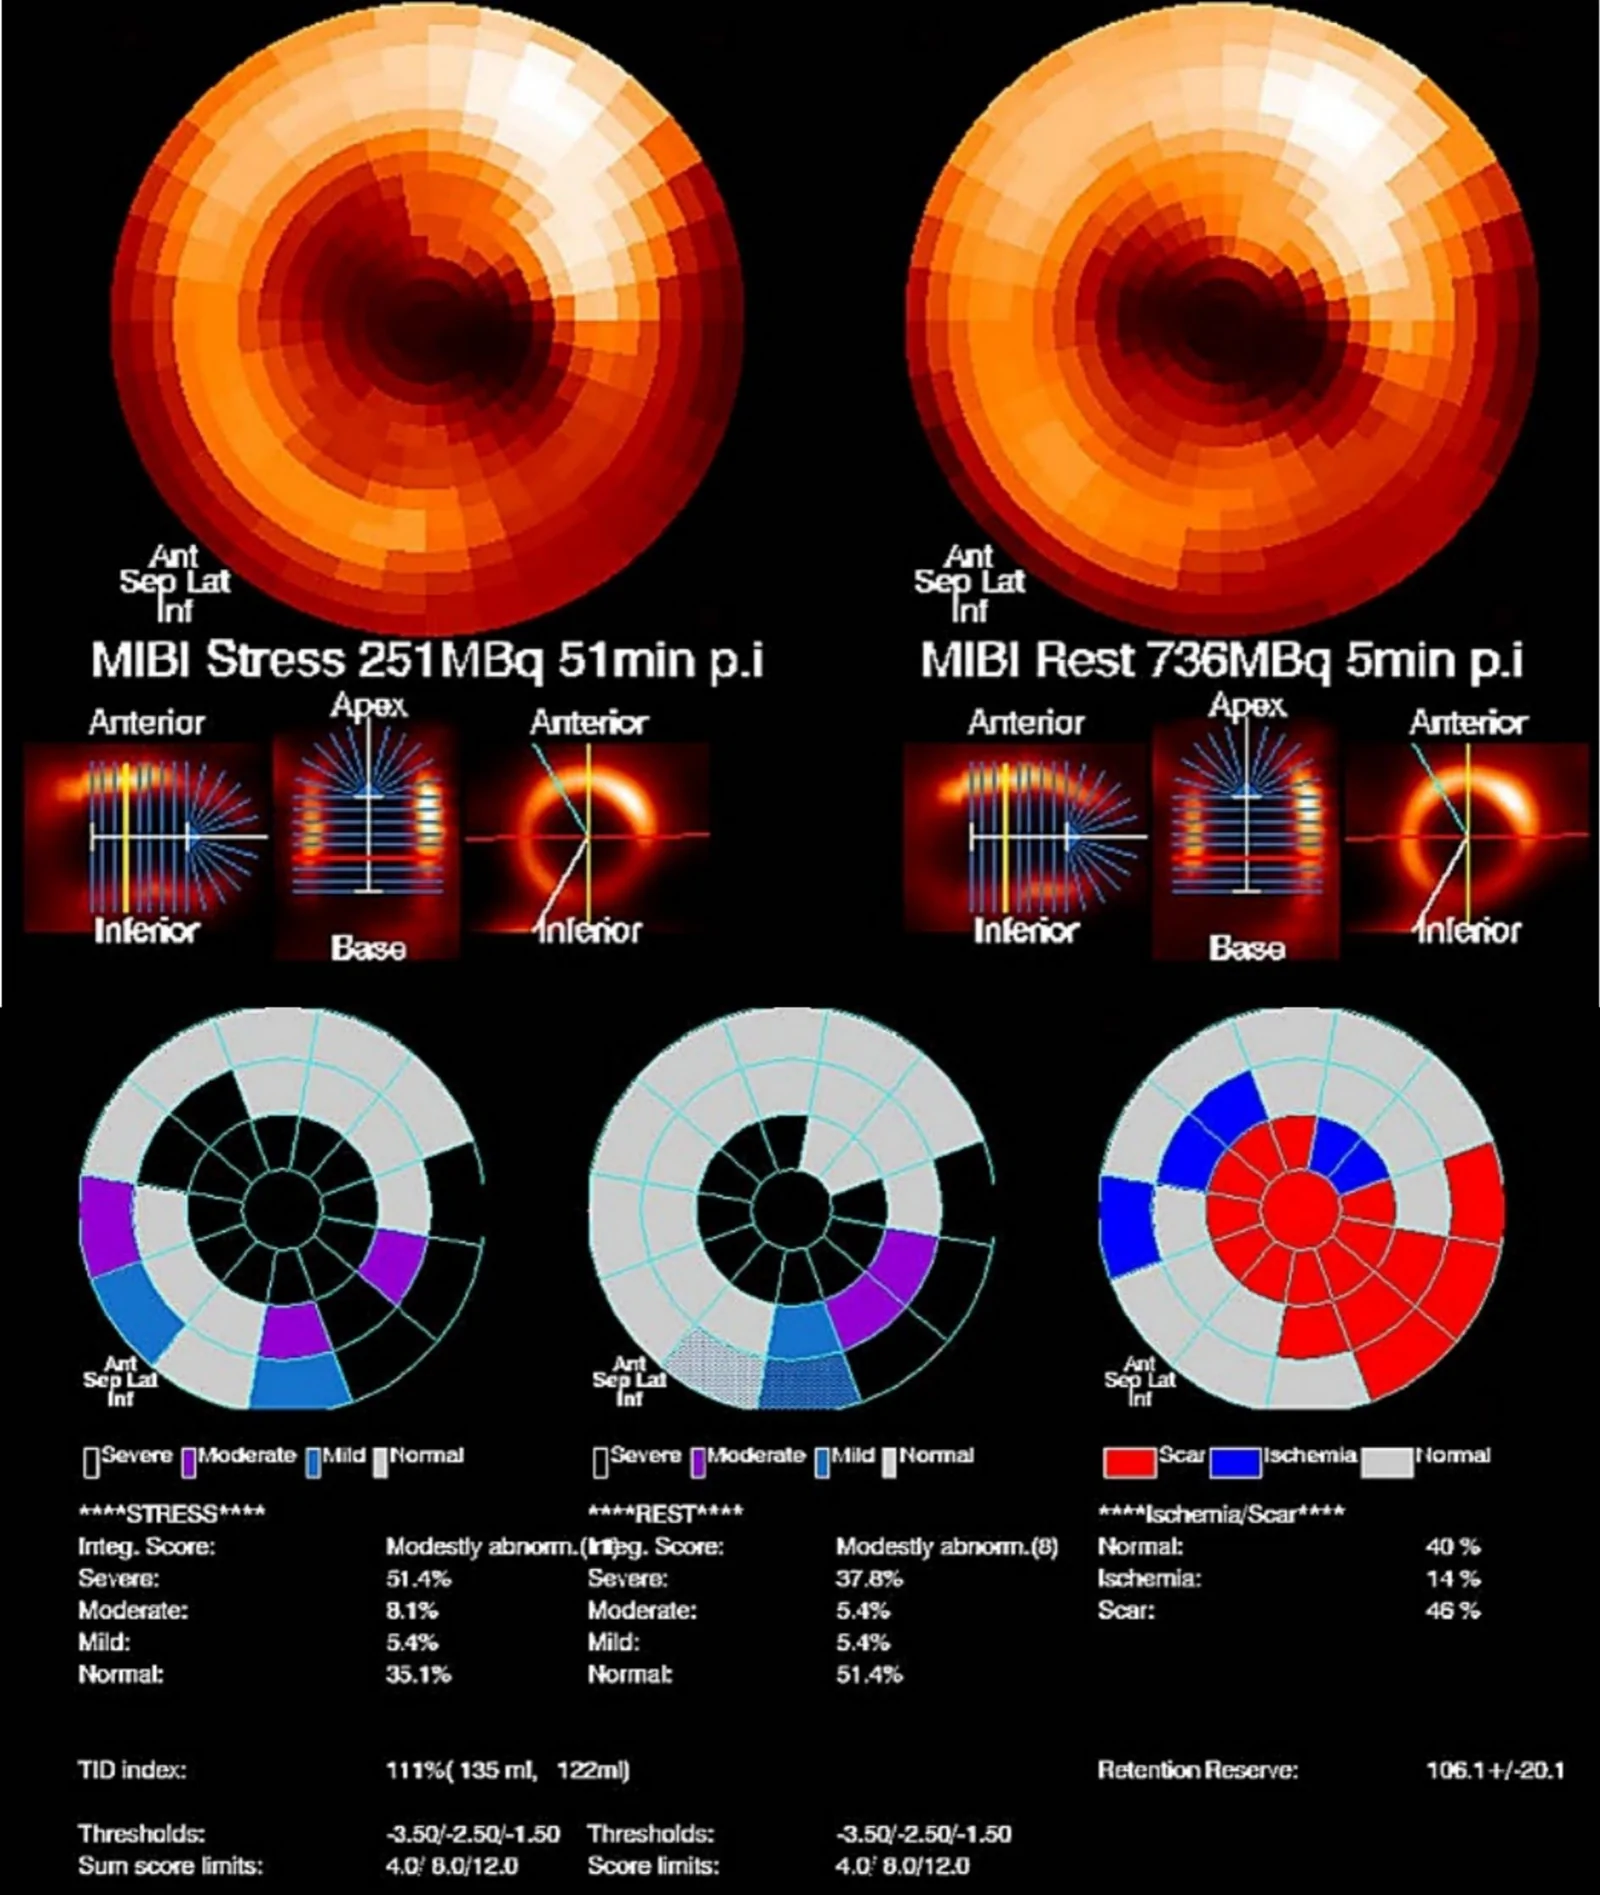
TPD analysis using software MunichHeart. Above: stress (left) and rest (right) perfusion polar maps, including their original LV SPECT images. Below: per-segment deficit severity score (0-3) and extent (%) for stress (left) and rest (middle) polar maps (black/purple/blue/gray: severe (3) / moderate (2) / mild (1) / normal (0) region). Right: according ischemia and scar segmentation (blue/red).
